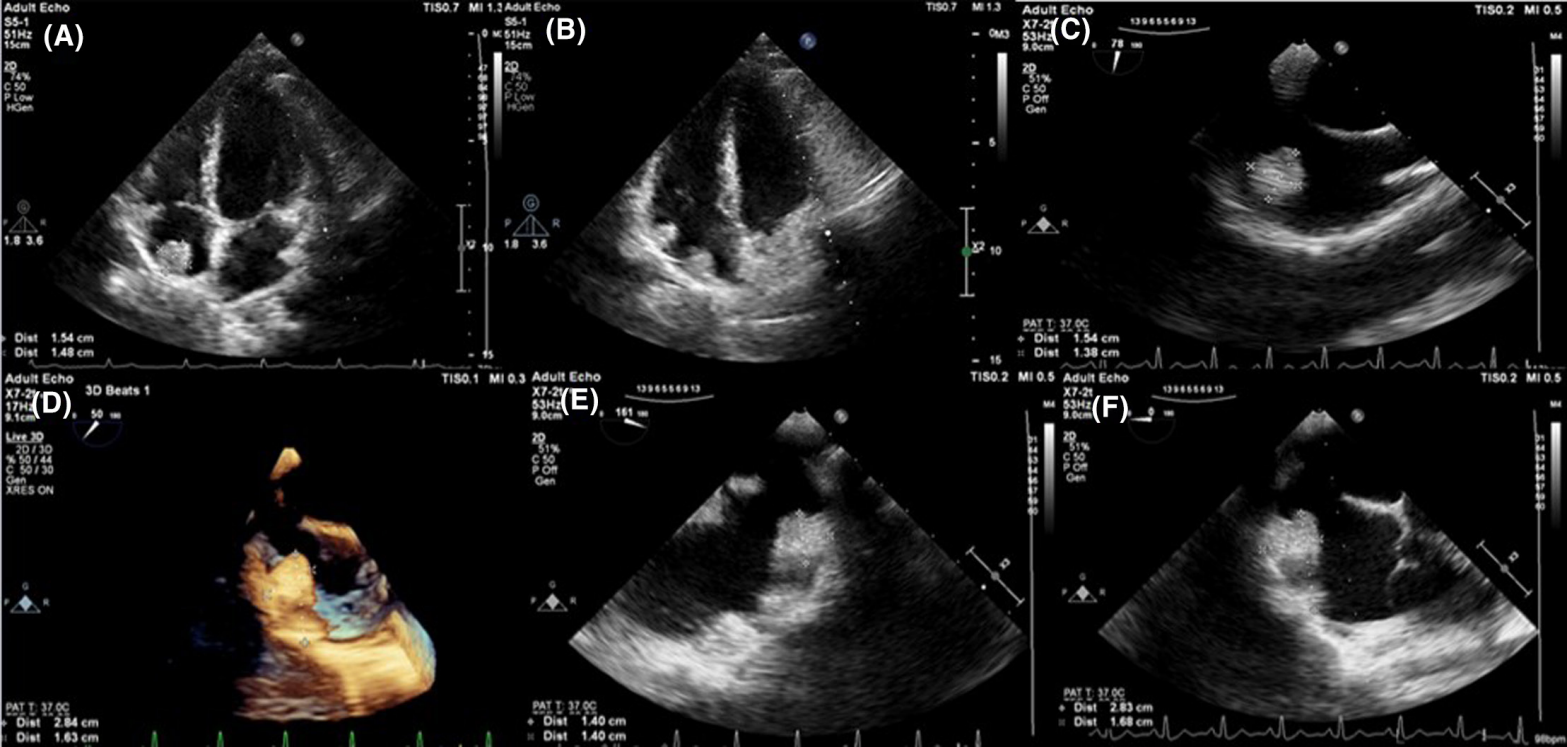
(A and B) Transthoracic echocardiography, (C–F) transesophageal echocardiography.
